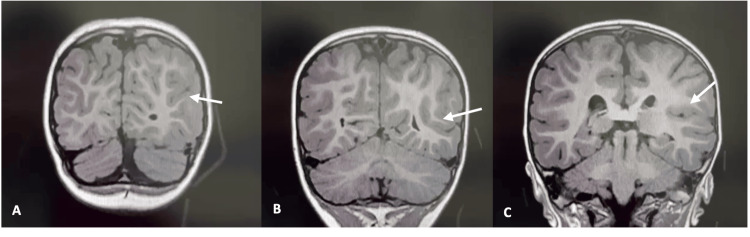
MRI findings of the right fronto-opercular and insular region. (A) Axial MRI slice showing an abnormally deep sulcus in the right fronto-opercular cortex (arrow). (B) Blurred gray-white matter interface in the right insular region, consistent with focal cortical dysplasia (arrow). (C) Enlarged view highlighting atypical sulcal orientation in the right opercular area (arrow).
